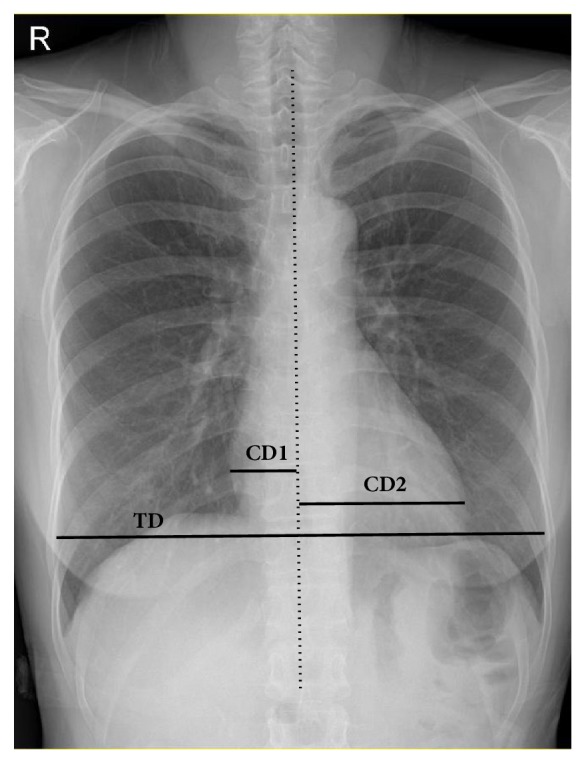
Measurement of cardiac diameter (CD) and thoracic diameter (TD). On chest PA, a vertical line (dotted line) was traced parallel to the vertebral column. The greatest distances from this line to each cardiac border (CD 1 and CD 2) were summed up to get CD. TD was defined as the greatest width (TD) between the inner surfaces of ribs. CD, cardiac diameter; TD, thoracic diameter; chest PA, posteroanterior chest X-ray.
